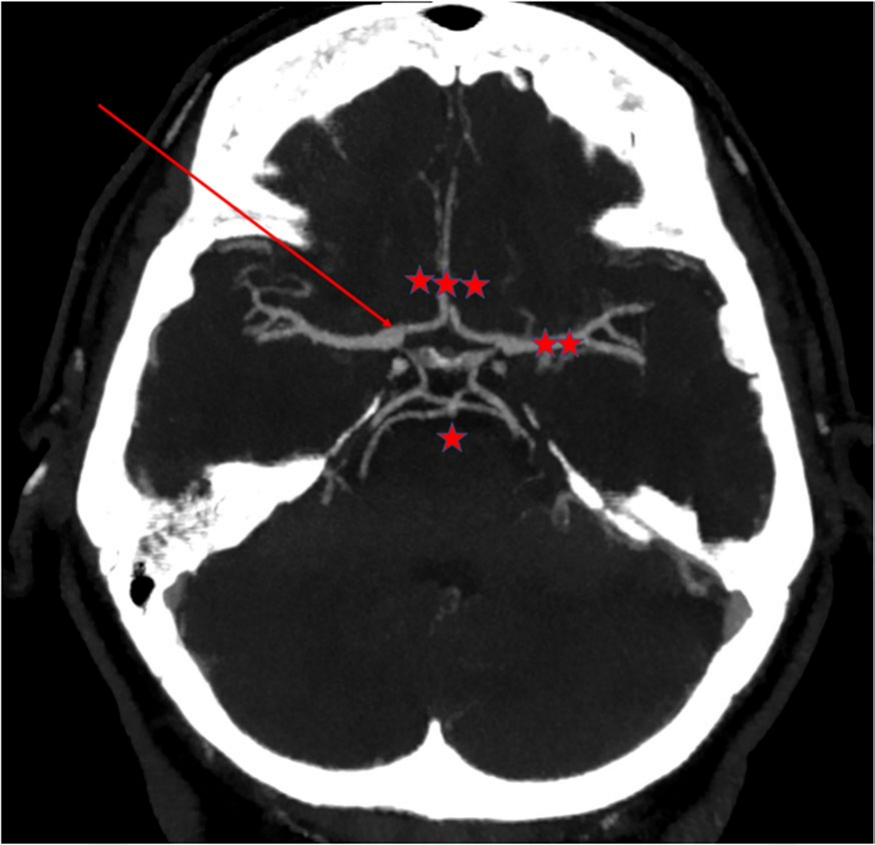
The CT angiography shows the circle of Willis (CW), which is the primary collateral system of the patients with steno-occlusive disease of the carotid. The red arrow signs the right internal carotid artery. It branches the medial cerebral artery (two red asterisks), the anterior cerebral arteries and the anterior communicant artery (three red asterisks), which constitute the anterior circle of CW. The one red asterisk signs the basilar artery, which branches the posterior cerebral artery and the posterior communicant arteries creating the posterior circle of CW
